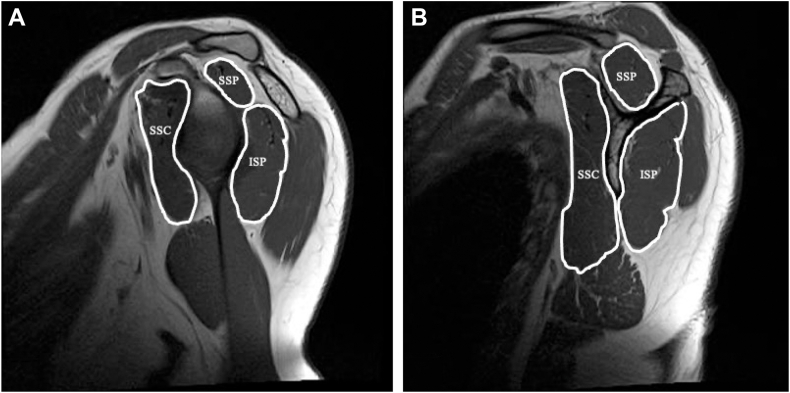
Measurement of muscle cross-sectional areas of the supraspinatus, infraspinatus, and subscapularis muscles on T1-weighted magnetic resonance images at the glenoid face and scapular spine levels. (A) Glenoid face level. (B) Scapular spine level. *ISP*, infraspinatus muscle; *SSC*, subscapularis muscle; *SSP*, supraspinatus muscle.
